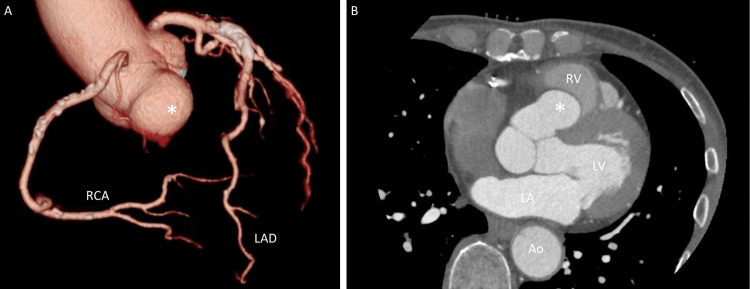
Computed tomography Three-dimensional reconstruction showed an aneurysm of the right sinus of Valsalva (A: asterisk). Note that there is no leakage of contrast medium from the aneurysm (B: asterisk) to the right ventricle (RV) on the axial image Ao, aorta; LA, left atrium; LAD, left anterior descending coronary artery; LV, left ventricle; RCA, right coronary artery
